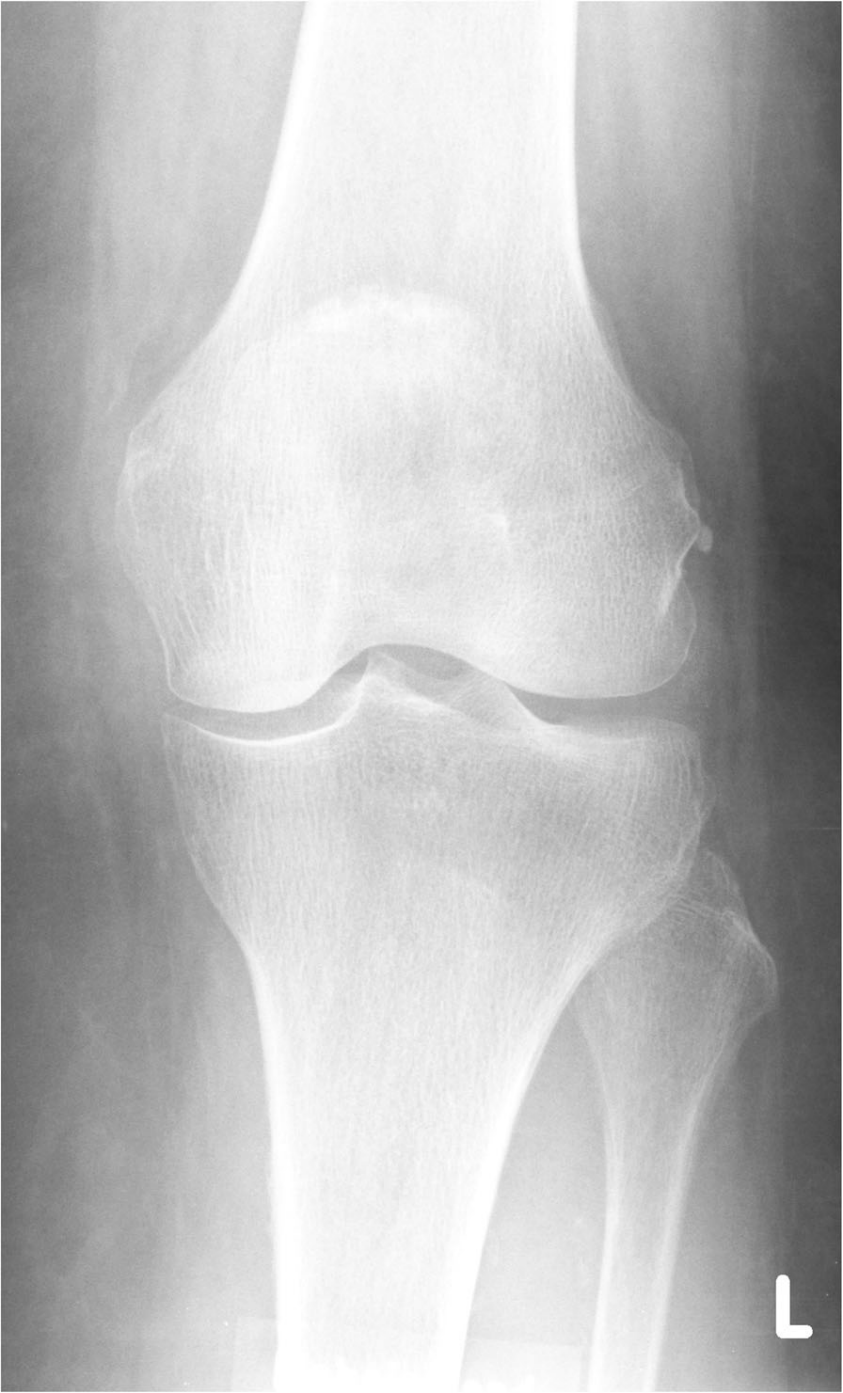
Case 1 patient: anterior-posterior radiography left knee: minimal medial joint space narrowing, absence of tibial osteophytes
